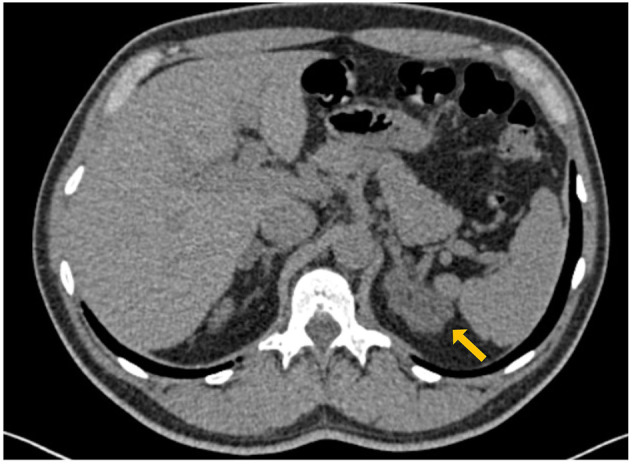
Abdomen CT scan showing bilateral enlargement of adrenal glands with a typical macro-nodular aspect of left adrenal (maximum diameters were 45 and 16 mm at left and right side with low Hunsfield Unit density (HU −20/+12), respectively).
